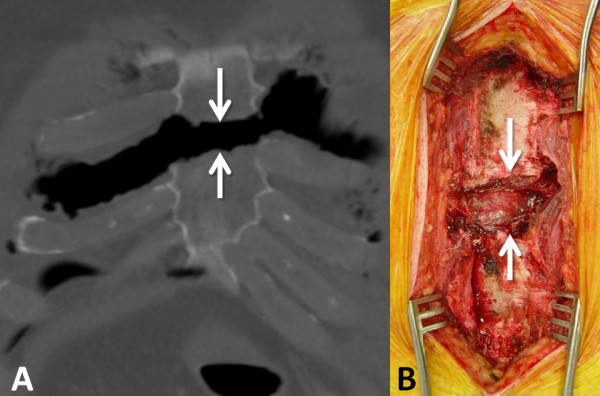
Displaced transverse sternal fracture in coronal CT scan (A) and operative site (B) after exposure for the sternal fracture fixation procedure. The arrows point out the impressive fracture diastasis of about 3 cm, with the retrosternal pericardium exposed in panel B.
